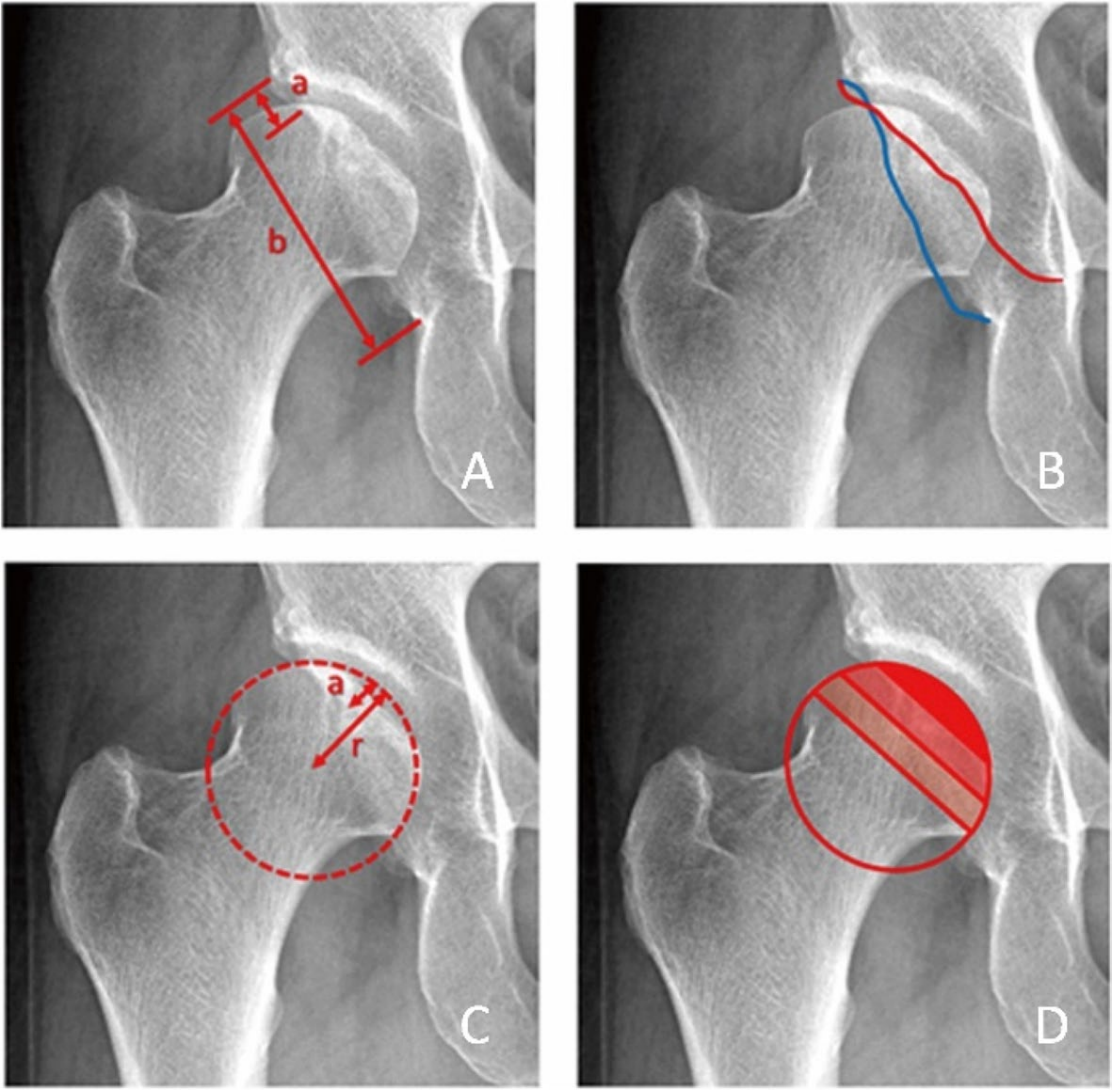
Four parameters for estimating the preacetabular anterior coverage were measured on standing pelvic AP radiographs. A. Crossover index: ratio of the width of the anterior acetabulum width (a) to the posterior wall width (b). B. Crossover sign: crossover between the anterior and posterior rims. C. AWI: The ratio of femoral head portion covered by anterior acetabulum (a) to femoral head radius (r). D. Rule of thirds: AWI 33%-66% is normal
